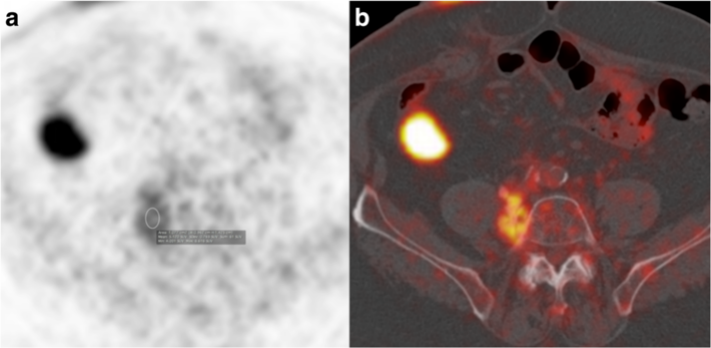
PET (a) and PET-CT fused axial slices (b) demonstrate the moderately hypermetabolic (SUV max 5.1) lesion of the retroperitoneum
